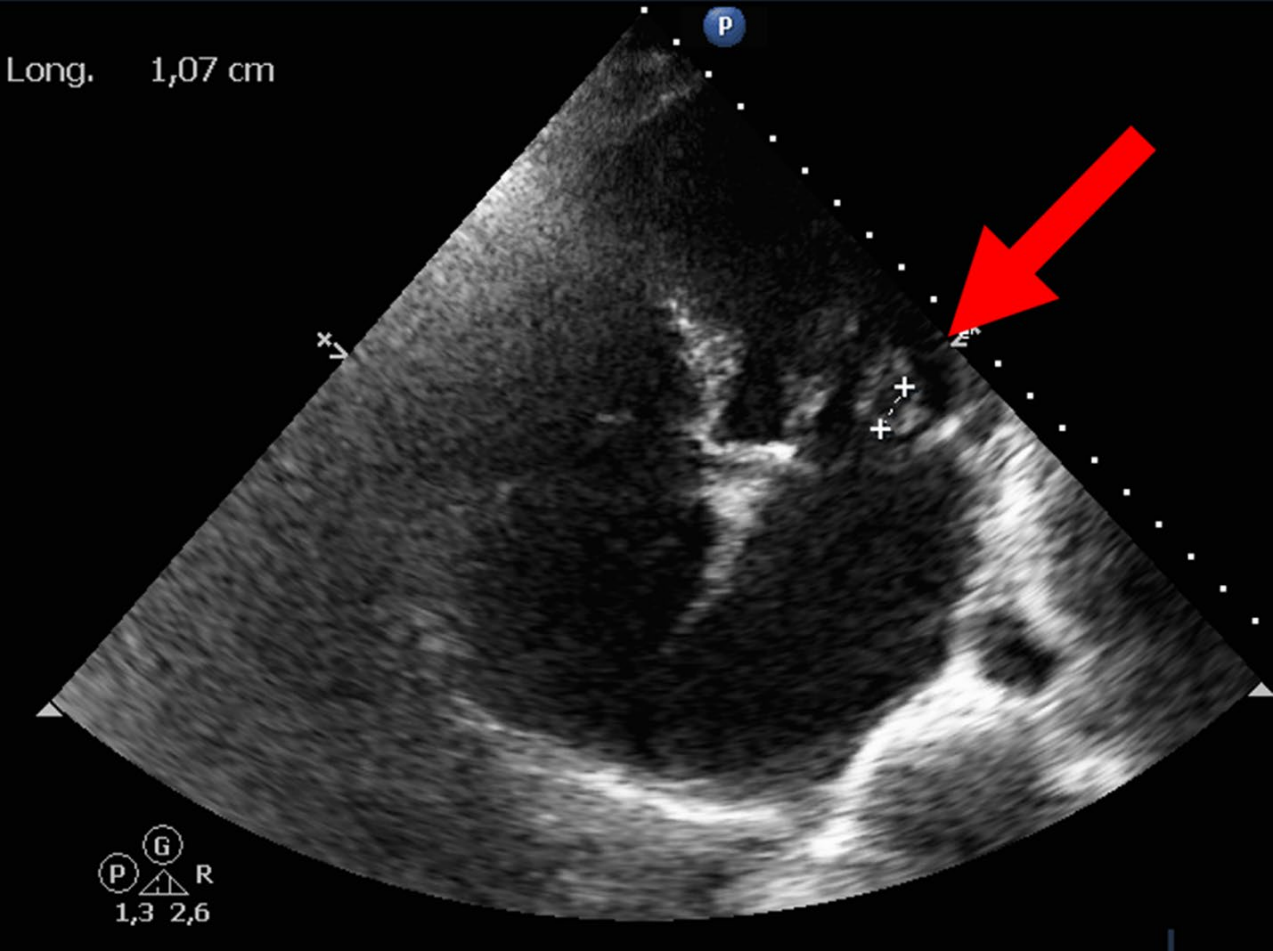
Transesophageal echocardiography. Transesophageal echocardiography, showing mobile echogenicity (*arrow*) on the small cusp of the mitral valve (prolapsing into the left ventricle).
